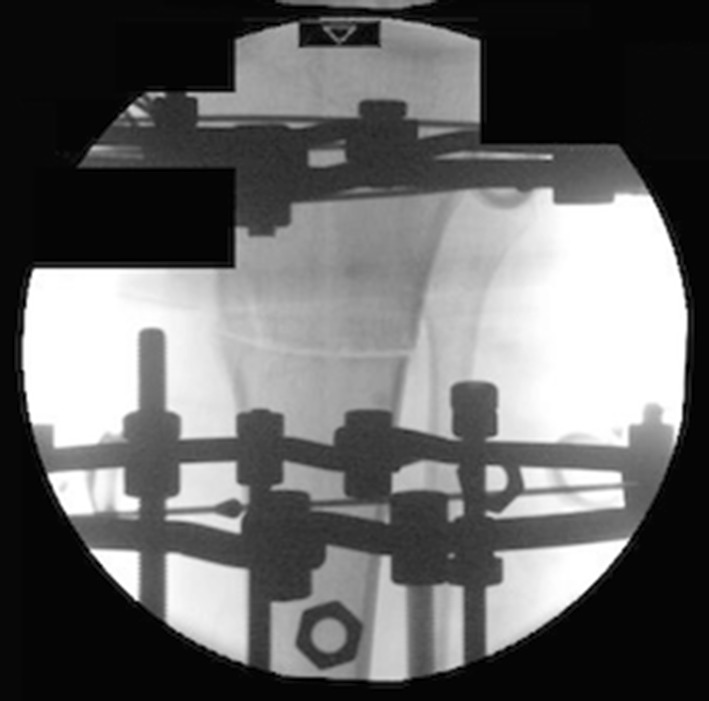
Clinical radiographs representing examples of the dome osteotomy in a proximal tibia with a circular frame in situ
